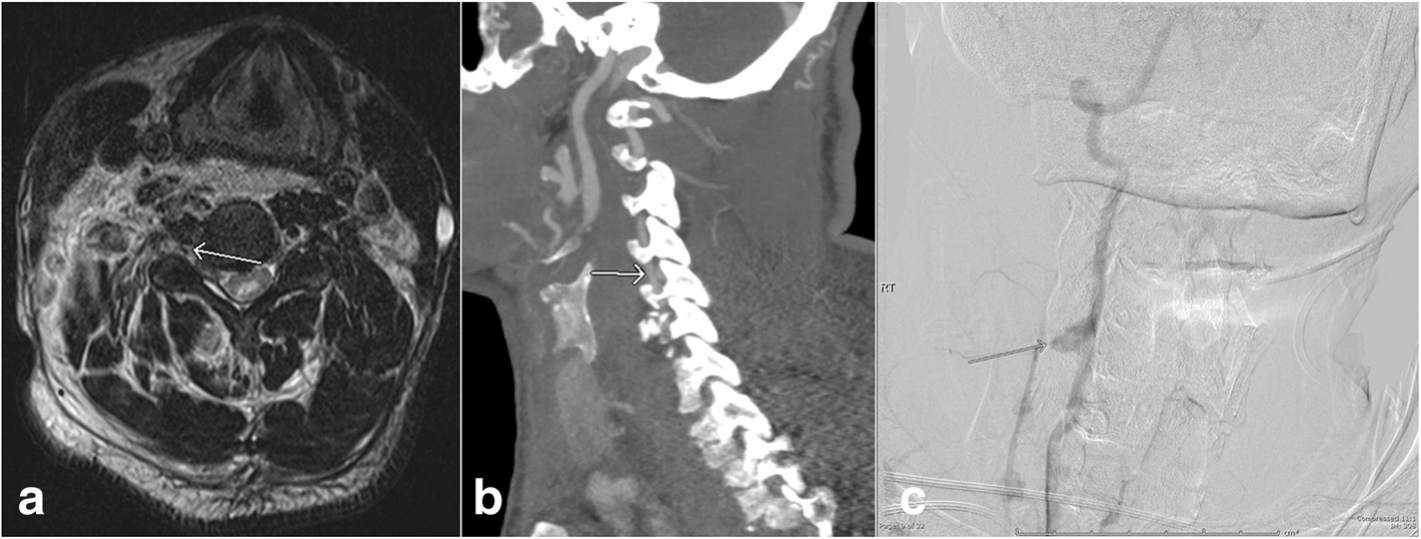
Axial T2 weighted image (a) shows the presence of post traumatic vertebral artery dissection with double lumen (*arrow*). Subsequent CT angiogram of the neck (b) confirms of the finding of vertebral artery injury (*arrow*). Follow-up angiography of the neck performed on the next day (c) shows the presence of pseudoaneurysm (*arrow*)
